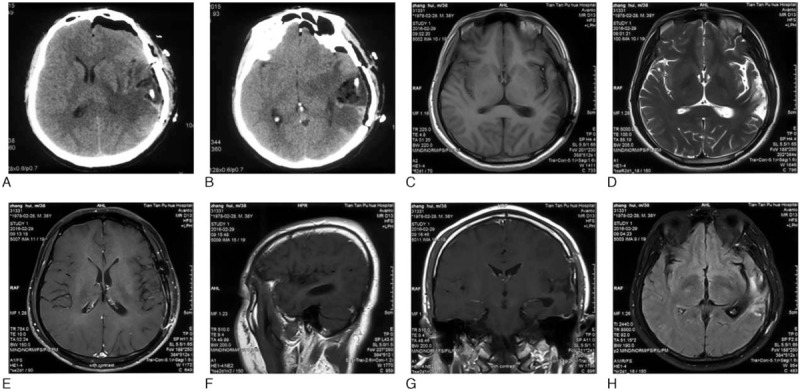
Postoperative brain CT and MRI images. (A and B) Axial view, CT images 1 day after surgery. (C–H) Nearly 5 months after surgery. (C) Axial view, T1-weighed image (T1WI). (D) Axial view, T2WI. (E) Axial view, contrast-enhanced T1WI. (F) Sagittal view, T1WI. (G) Coronal view, contrast-enhanced T1WI. (H) Axial view, diffusion weighted imaging (DWI).
